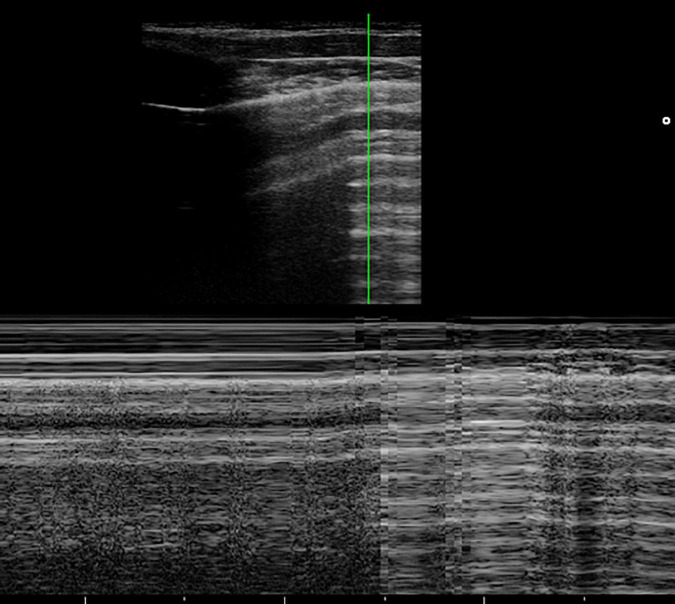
The grayscale lung ultrasound examination (transverse scan between the intercostal fields; linear probe with a frequency of 12 MHz) of a 15-year-old boy with left apical pneumothorax shows the *Barcode sign* identified in M-mode as a transition from the normal lung (*linear granular pattern on the right of the figure*) to the pneumothorax (*linear pattern on the left of the figure*). The grayscale lung ultrasound examination (transverse scan between the intercostal fields; linear probe with a frequency of 12 MHz) of a 16-year-old boy with right apical pneumothorax, shows the *Lung Point sign* identified in B-mode as the point where normal sliding disappears due to the presence of air in the pleural cavity.
